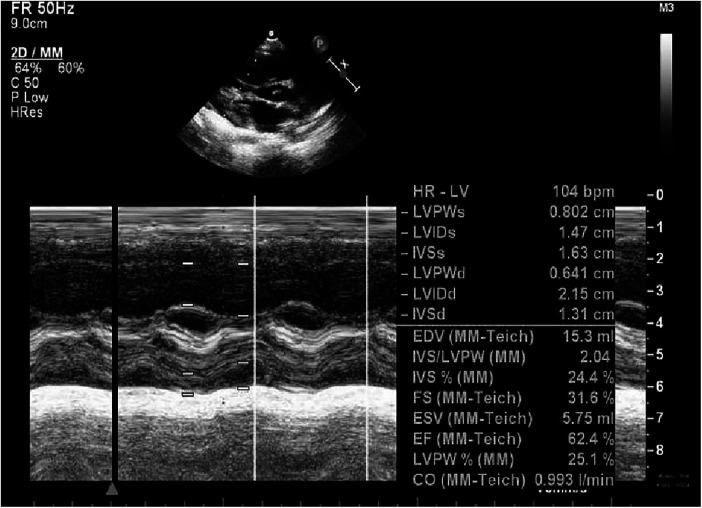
Echocardiography at 1 m.
